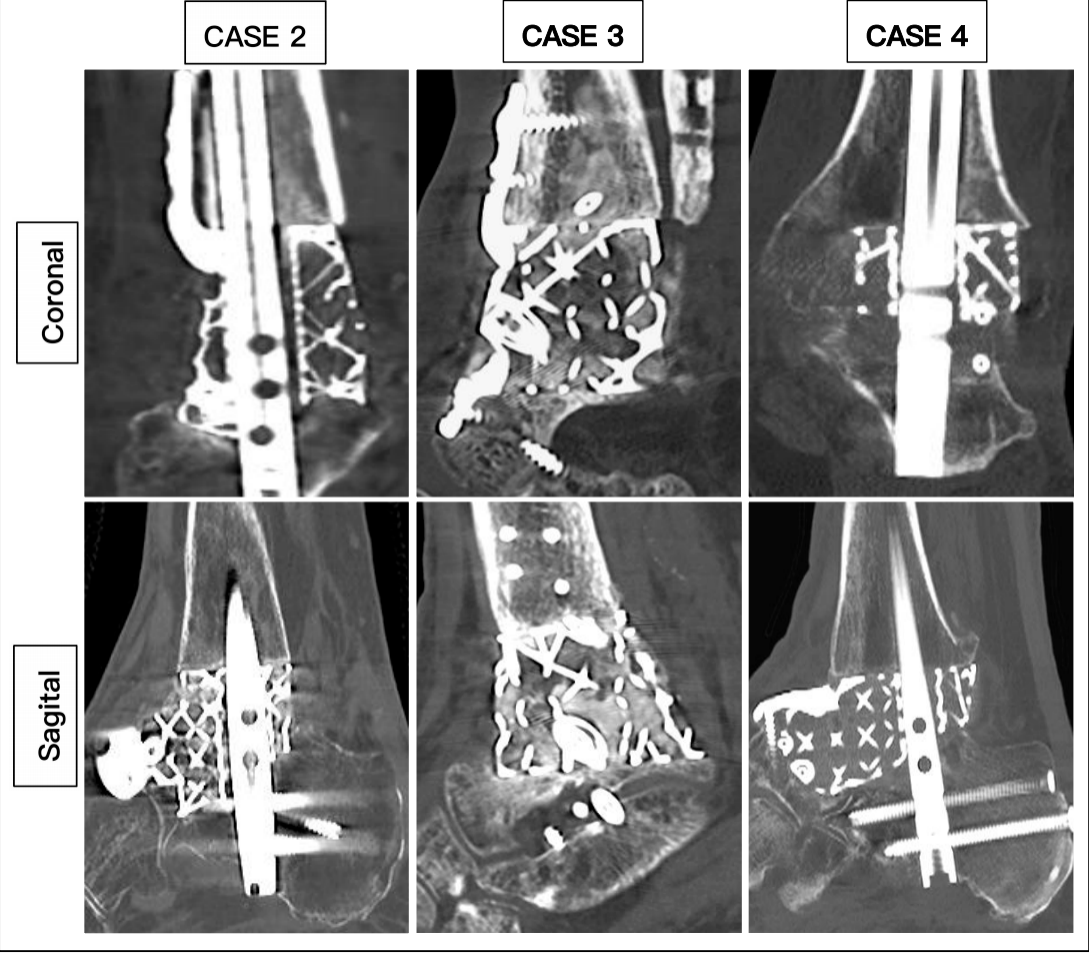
Demonstration of union from CT scans.
